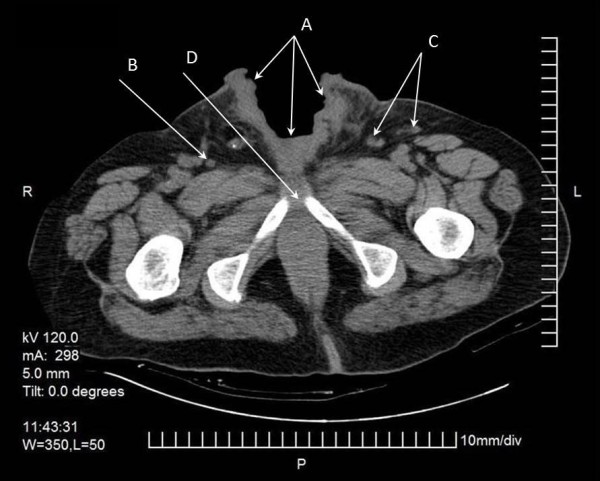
Abdominal image from computed tomography (CT) scan. The chasm of the lower abdominal wall (arrow A) and the inguinal lymph nodes bilateral can be identified (arrows B and C). The abdominal CT scan also showed lymph nodes of pathological numbers and sizes bilateral in the iliac vessels and inguinal areas, as well as an erosion of the pubic bone (arrow D).
